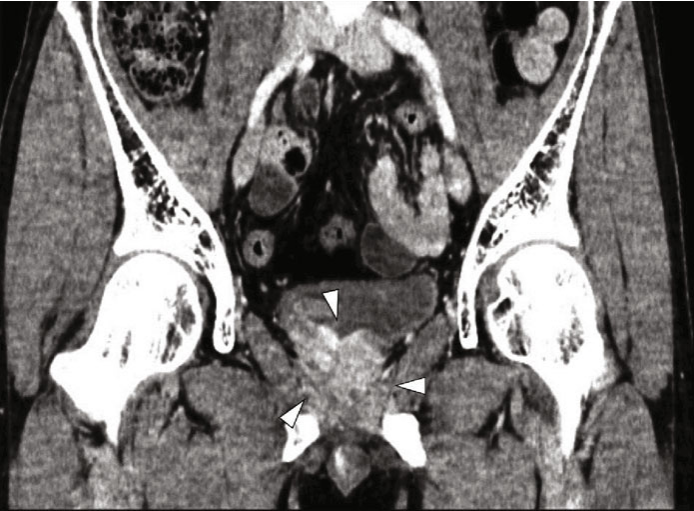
Prostate size was obviously reduced based on computed tomography after the administration of docetaxel (arrows).
